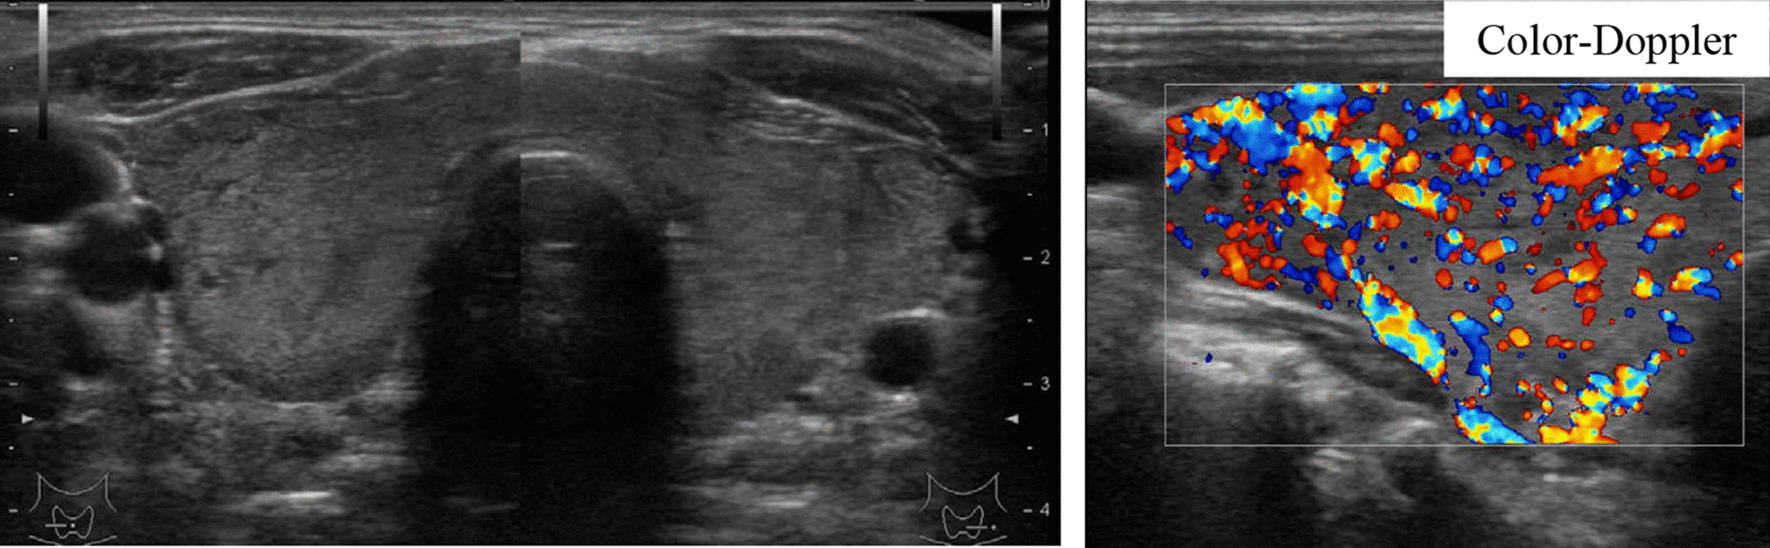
Thyroid ultrasonography showed diffuse enlargement of both lobes with heterogeneous echogenicity. No tumor lesions were detected. Neither lobe showed increased blood flow on Color-Doppler imaging
